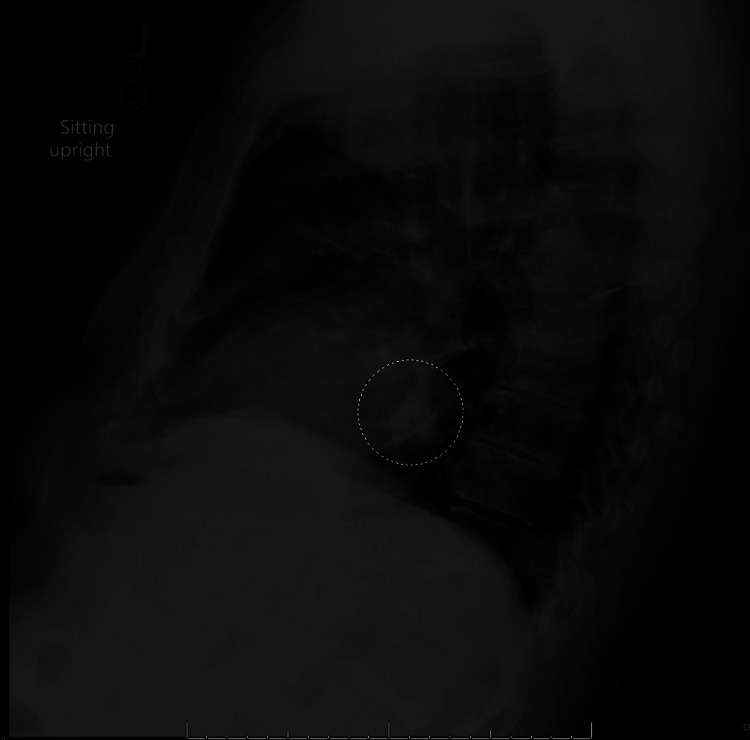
Chest X-ray with 2.5 cm infrahilar nodule
